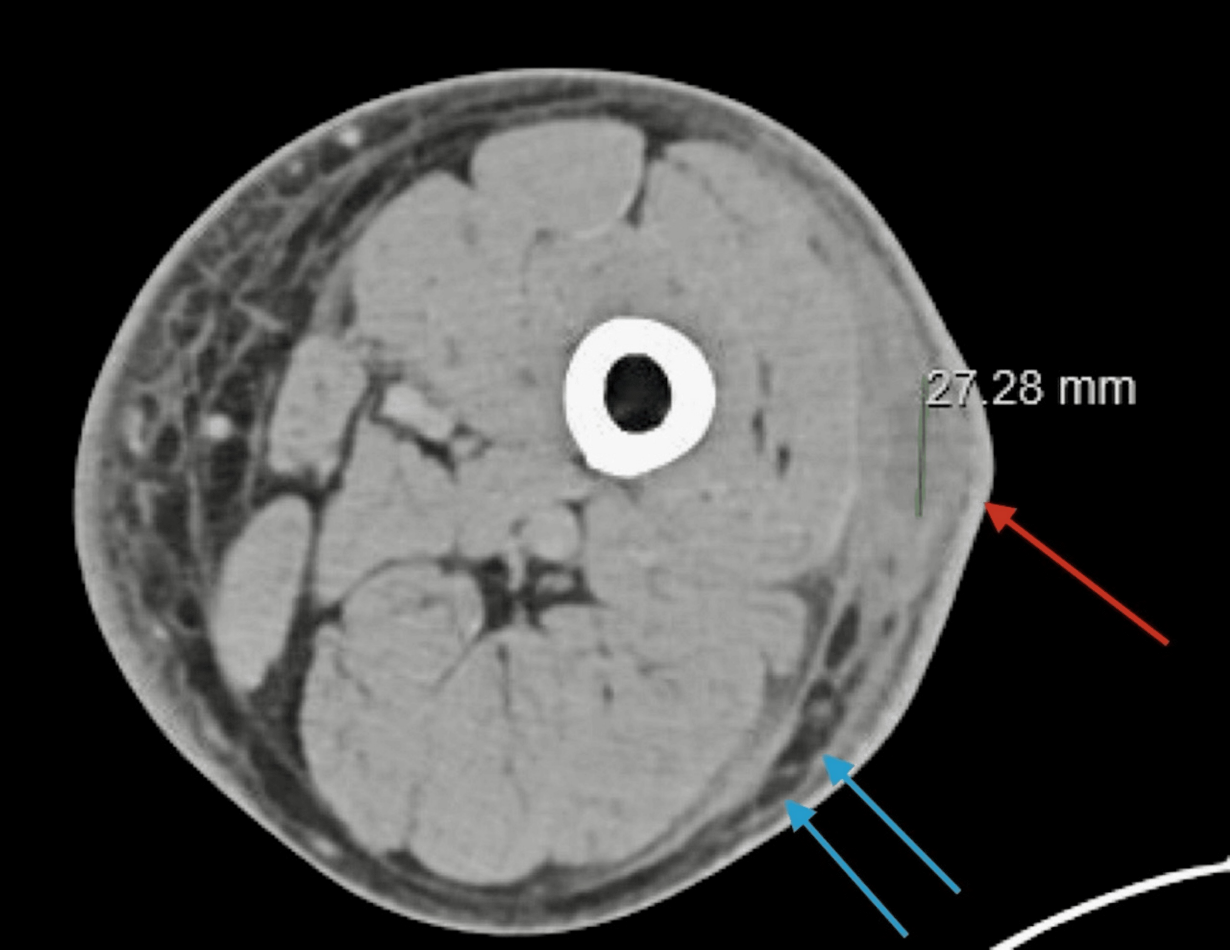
CT impression: Diffuse subcutaneous edema in the thigh. There is a superficial fluid collection in the mid-lateral thigh, approximately 17 cm cranial to the joint space measuring up to 2.7 x 5.5 x 2.1 cm (red arrow). There is also fluid tracking inferiorly toward the level of the knee joint in the posterior lateral subcutaneous tissues measuring up to 3.9 x 12.0 x 2.3 cm (blue arrow). Findings likely reflect abscess in the setting of clinical concern for cellulitis.
